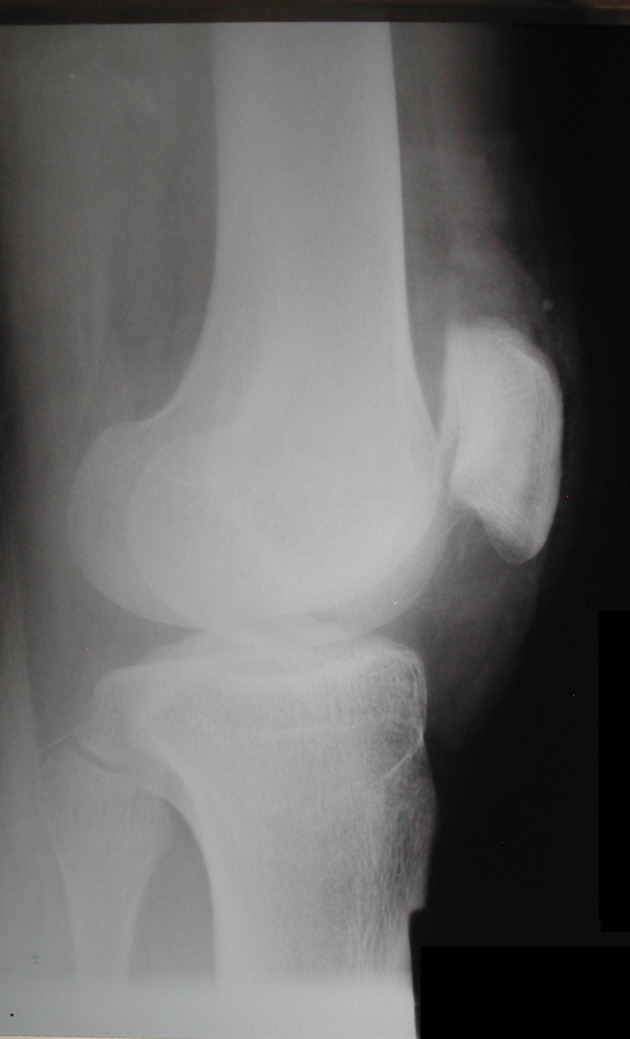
Lateral radiograph demonstrated the fracture by the articular gap in patella. Note the difference between the lateral and the medial side of patella caused by the displaced vertical trace of fracture.
